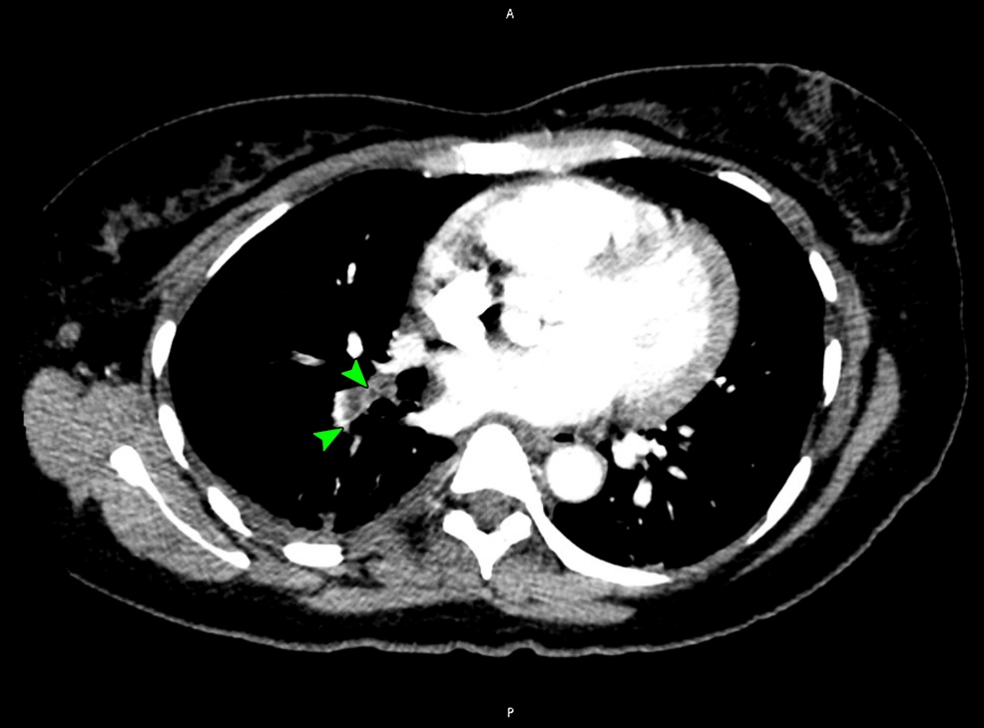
A CT pulmonary angiogram image showing a large right pulmonary embolus extending into the right lower lobe segmental branches (arrowheads) CT: computed tomography
